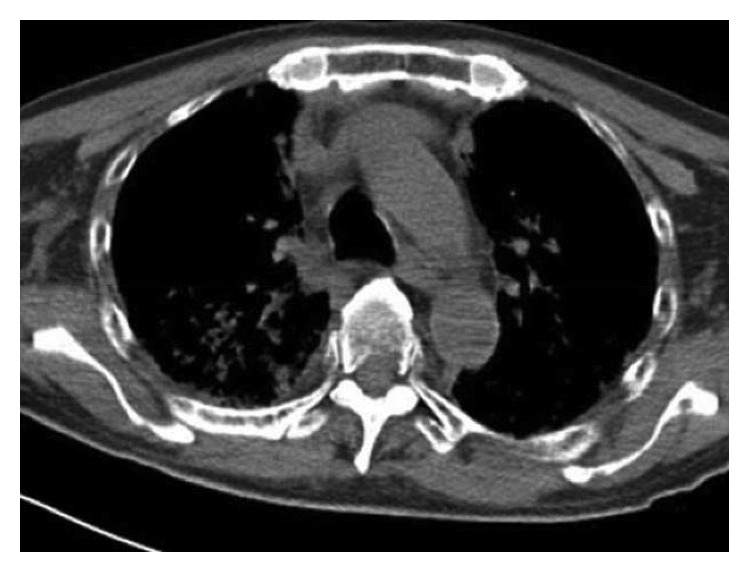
CT chest showing new interval appearance of bilateral perihilar ground-glass opacities and peribronchial and interstitial thickening predominantly in the upper lobes.
